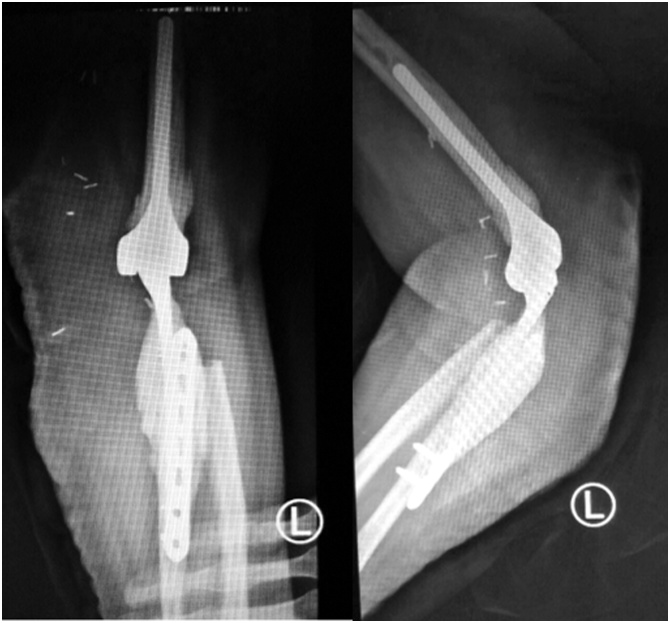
Post Operative Xray. Showing elbow arthroplasty, ulnar bone was reconstructed using a 3.5 locking plate.
